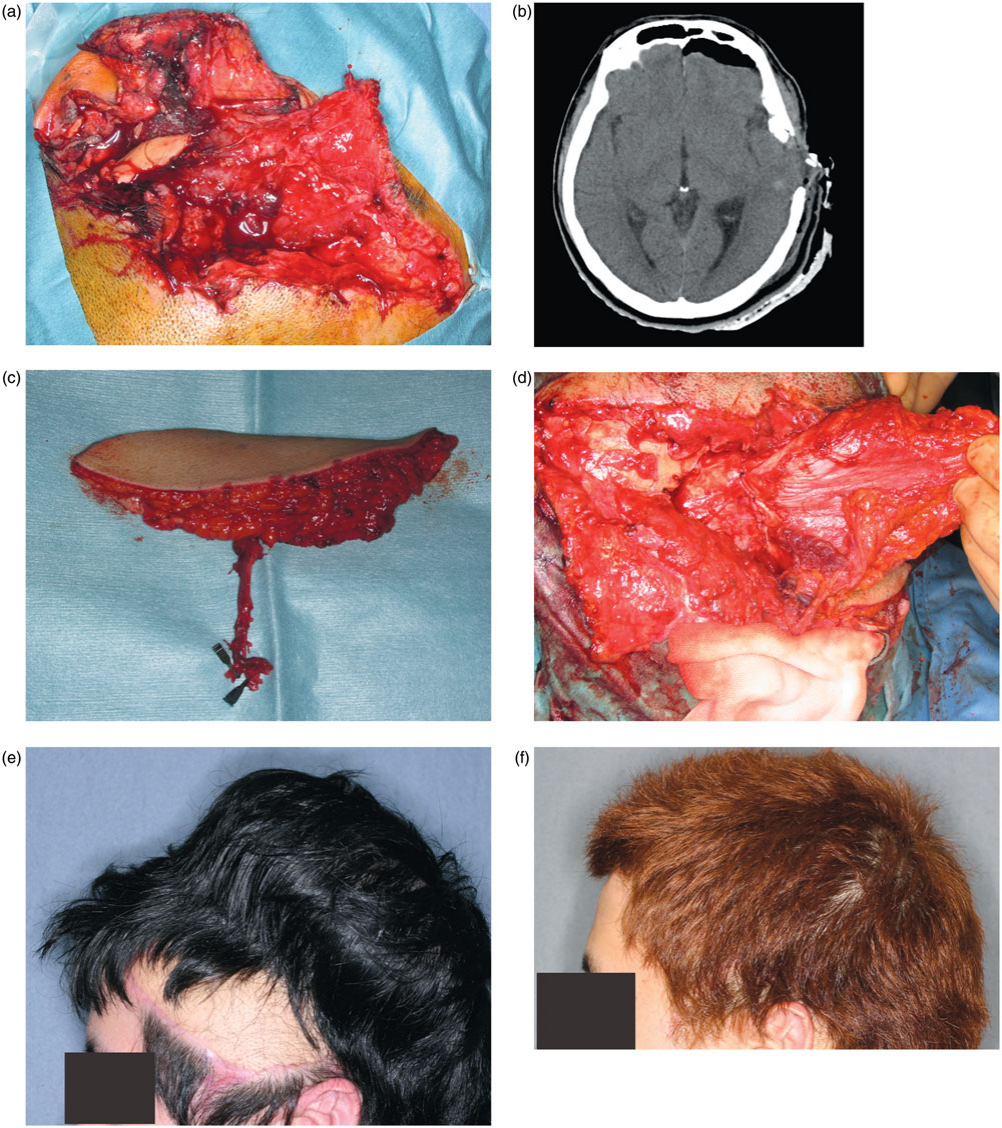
(a) The patient had a large defect (including the soft tissue, scalp, and dura) in the left temporal region. The wound was contaminated with sand, pieces of metal, and machine lubricant. (b) CT findings suggest that the depth of the wound reached the brain tissue. (c) A 15 × 8 cm anterolateral thigh flap with 8 × 8 cm of fascia was harvested. (d) Vascularized fascia was fixed to the edge of the dura. (e) A 70 × 120-cm tissue expander was inserted under the scalp. Preoperatively, 270 ml of expansion was achieved. (f) Two years after final surgery. The skin paddle made from the anterolateral thigh flap was completely removed.
